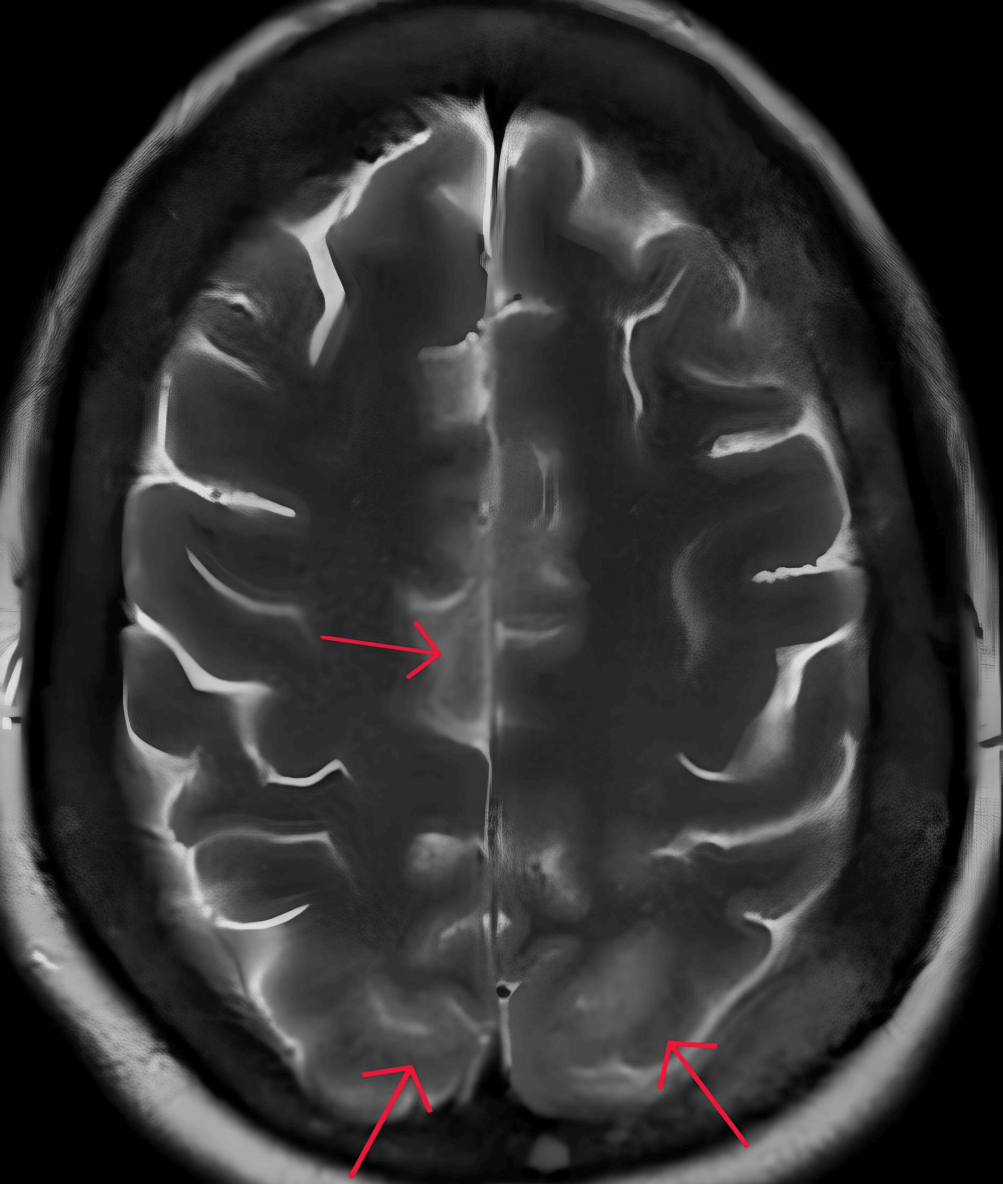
T2-weighted axial MRI slice Multi-focal area of high-signal intensity in the bilateral parieto-occipital lobes
